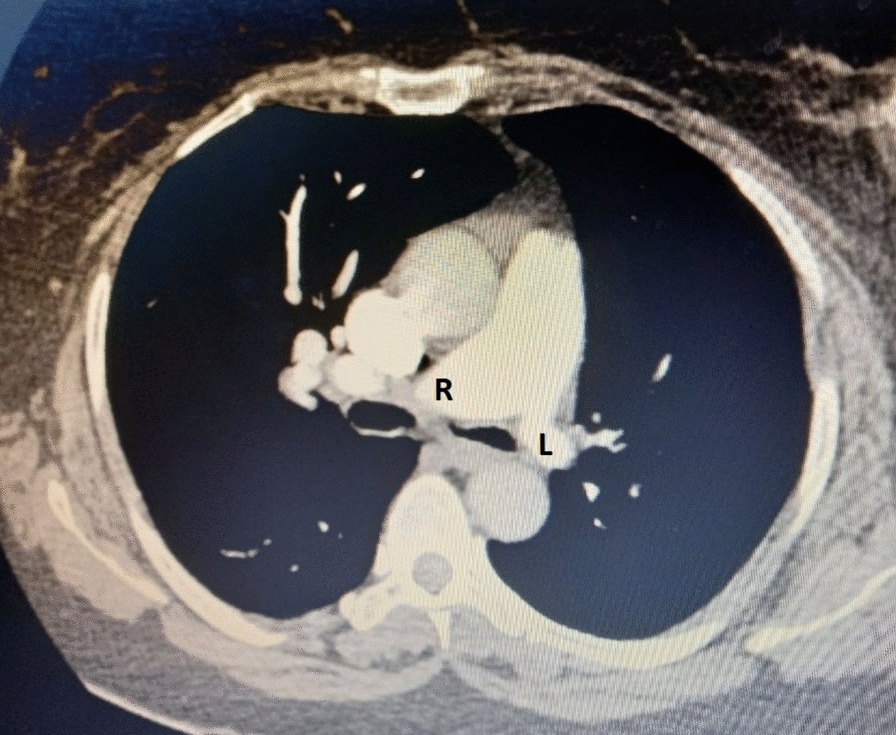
Chest angio-computed tomography (mediastinal window) displaying left pulmonary artery (L) hypoplasia
